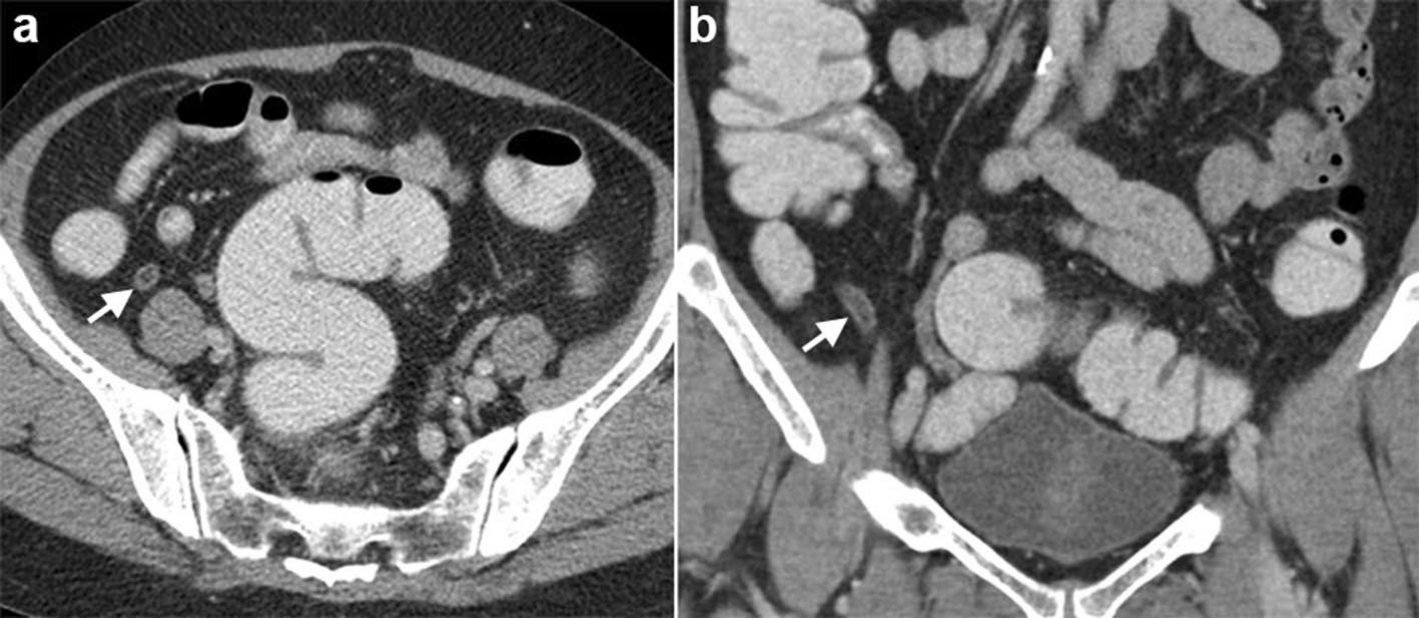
Acute appendicitis in a 65-year-old female with Alvarado score of 7. Cross-sectional (a) and coronal (b) computed tomography scan showing enlarged appendiceal diameter and wall thickening (arrows).
